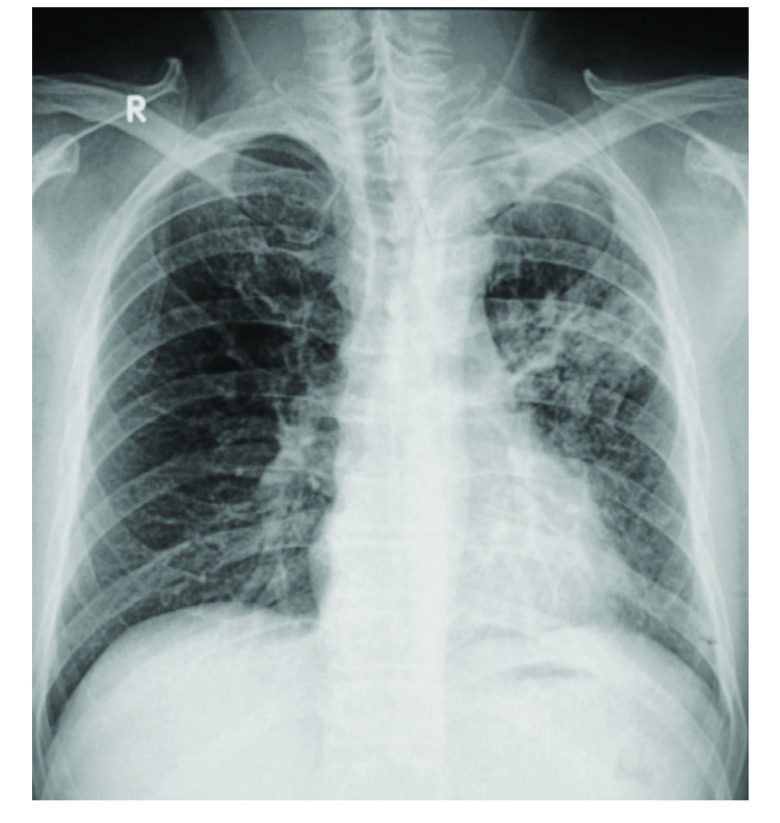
Repeat chest X-ray of a 64-year-old man done almost 3 months after the first X-ray, showing improvement
